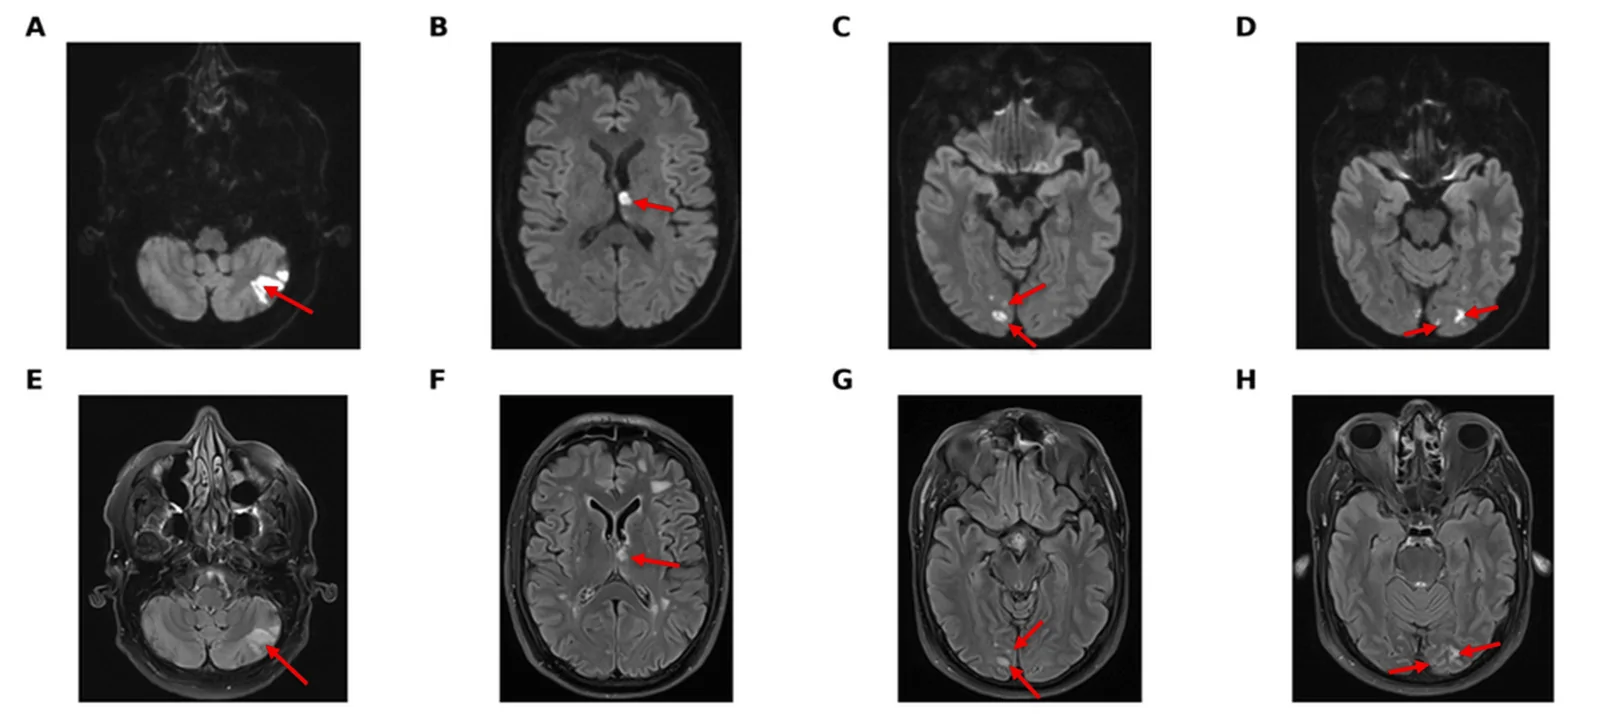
Brain magnetic resonance imaging demonstrating ischemic lesions confined to the posterior circulation. Diffusion-weighted images show lesions in the left cerebellum (A), left thalamus (B), right occipital lobe (C), and left occipital lobe (D). Corresponding FLAIR images show lesions in the left cerebellum (E), left thalamus (F), right occipital lobe (G), and left occipital lobe (H). The arrows indicate the ischemic lesions. DWI: Diffusion-weighted imaging; FLAIR: Fluid-attenuated inversion recovery.
